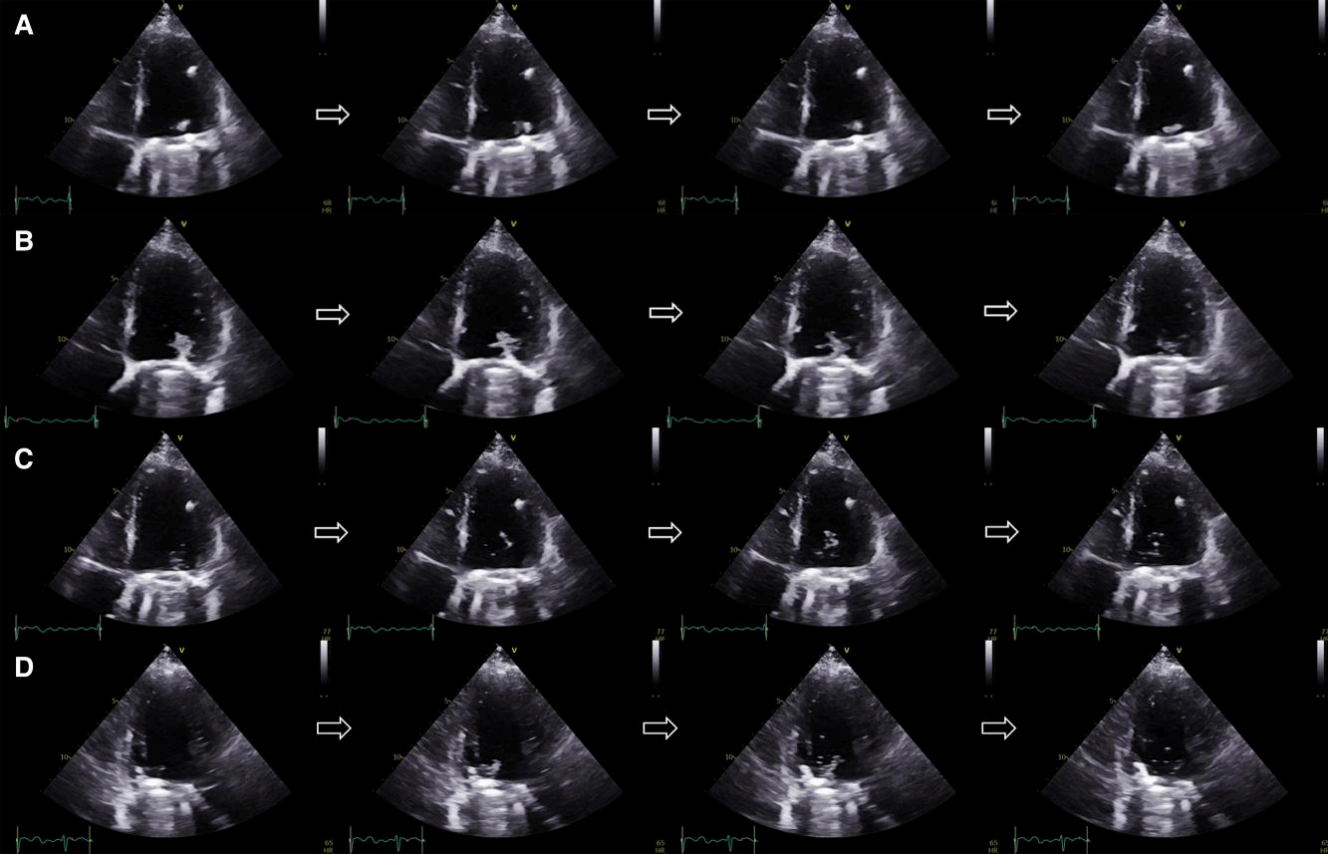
Transthoracic echocardiogram. (*A–C*) Apical four-chamber. (*D*) Apical three-chamber. (*A*) A mobile, echo dense mass in the ventricular surface of the mechanical mitral valve. (*B*) Changes in the size and shape of the mass. (*C* and *D*) The cluster of microbubbles and how they disappear.
